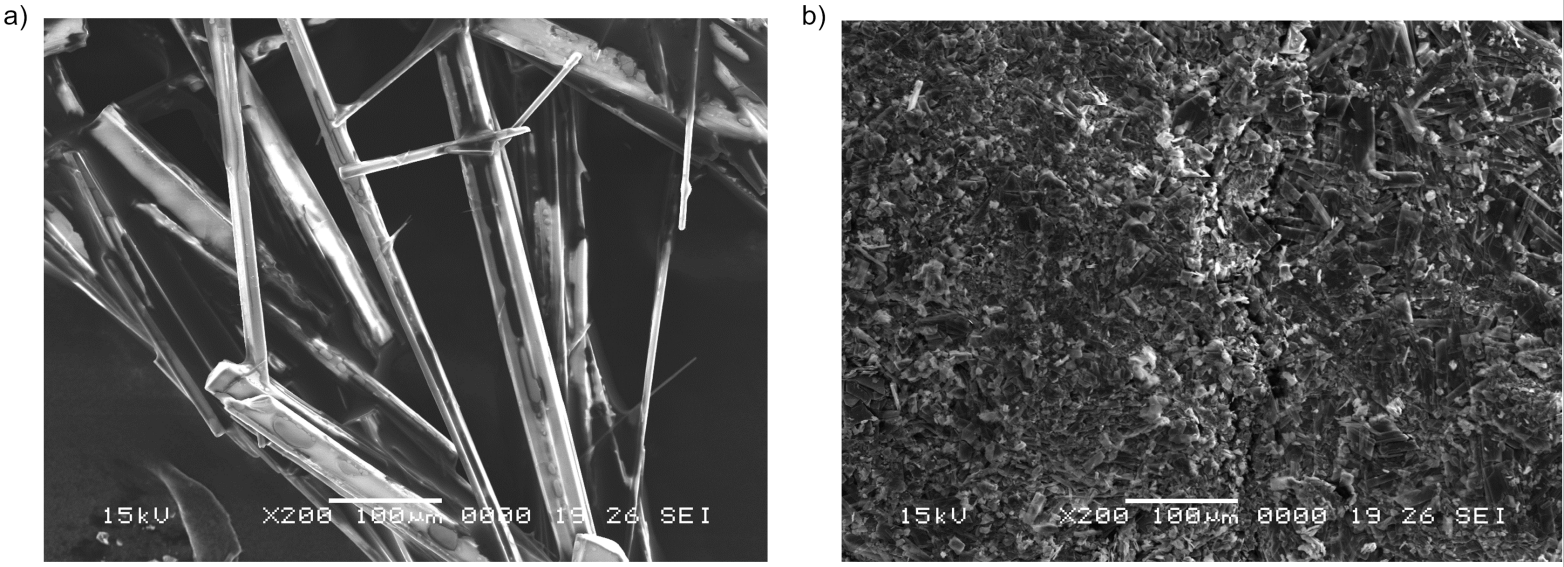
SEM image recorded at 200× for the raw reaction product 2c obtained through a) ultrasound-assisted reaction conditions and b) classical reaction conditions.
